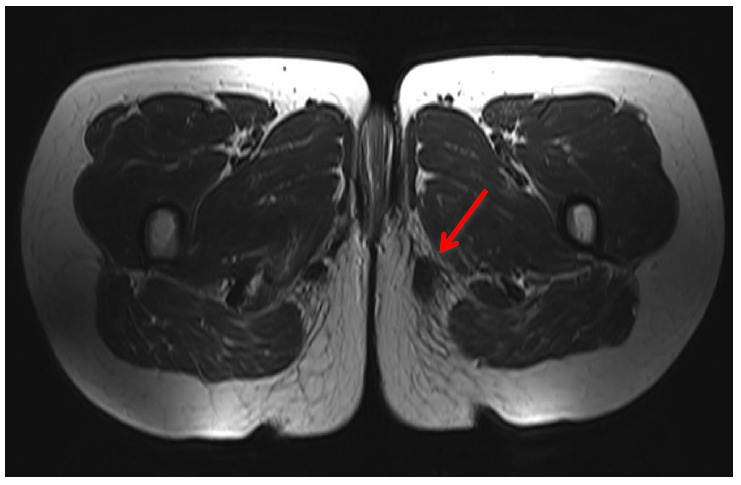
MRI pelvis - Axial T1 weighted image of the perineal region, demonstrating a low signal intensity lesion to the left of the midline in the superficial soft tissue of the perineum
